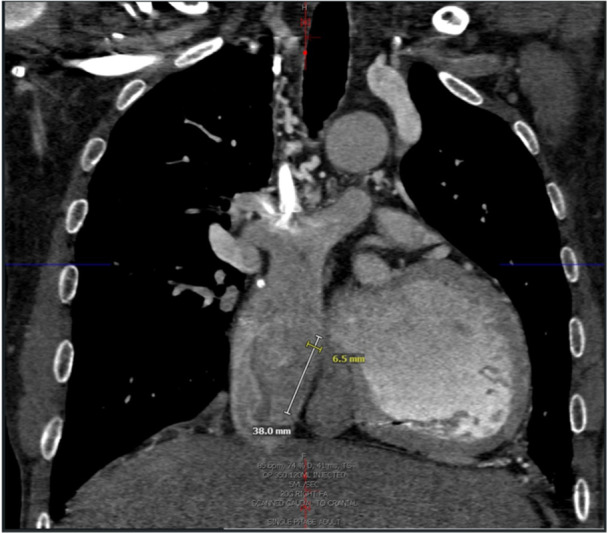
Computed tomography scan, anterior‐posterior view of the lateral tunnel Fontan with measurement length and width of the Aveir VR (38 mm by 6.5 mm).
